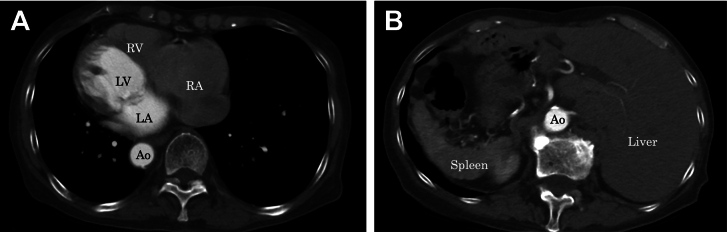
(A) Computed tomography imaging of the chest shows dextrocardia. (B) Computed tomography imaging of the abdomen shows situs inversus totalis. (Ao, aorta; LA, left atrium; LV, left ventricle; RA, right atrium; RV, right ventricle.)
